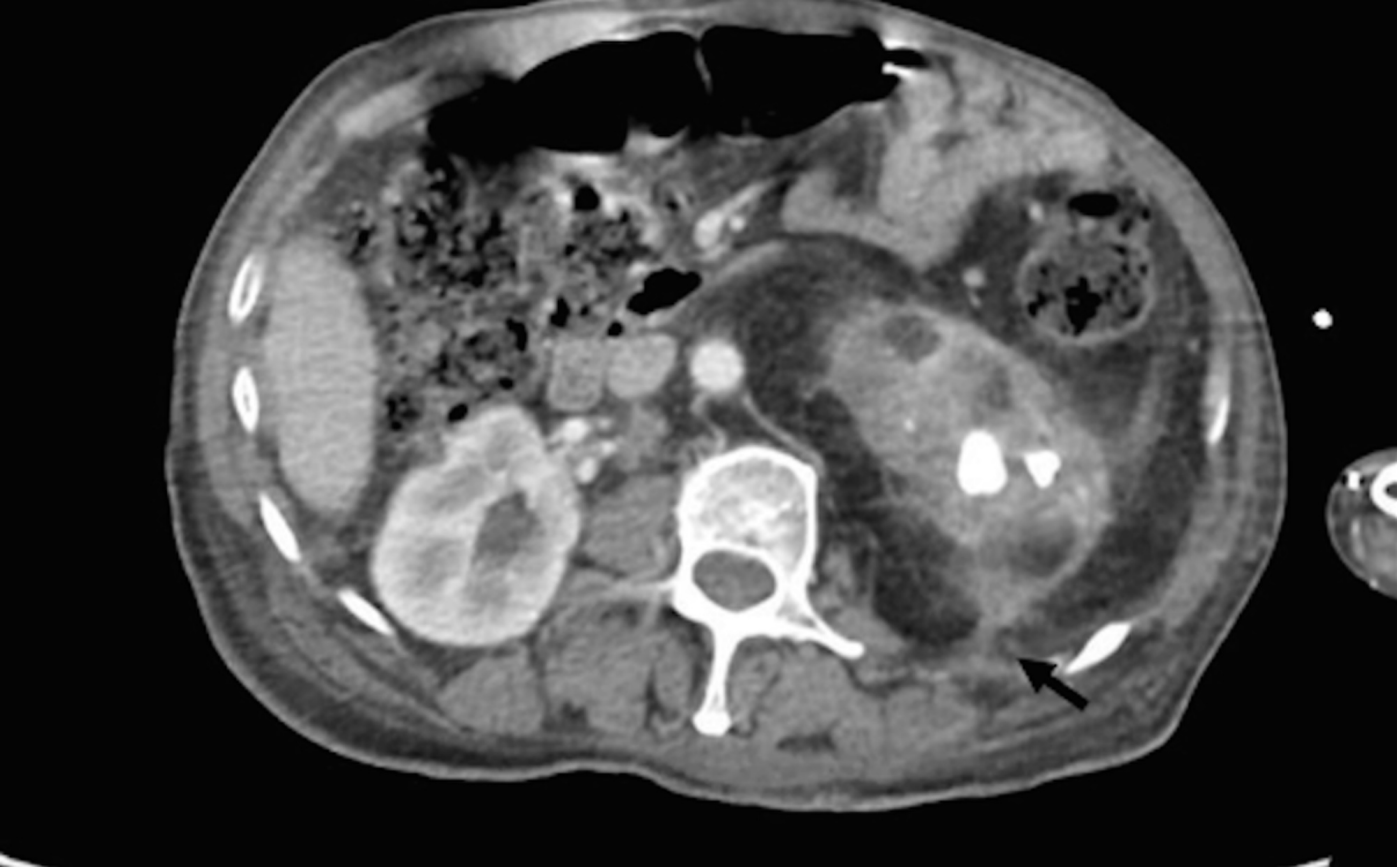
CT scan showing a fistulous tract involving the para-spinal muscles CT: computed tomography
